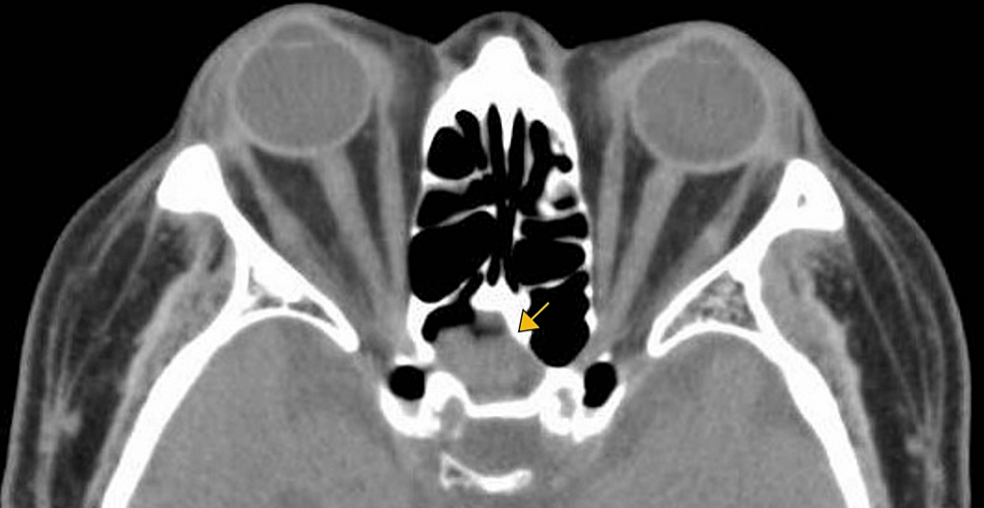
Computed tomography shows mild accumulation of fluid in the right posterior ethmoid sinus without bone destruction (arrow)
